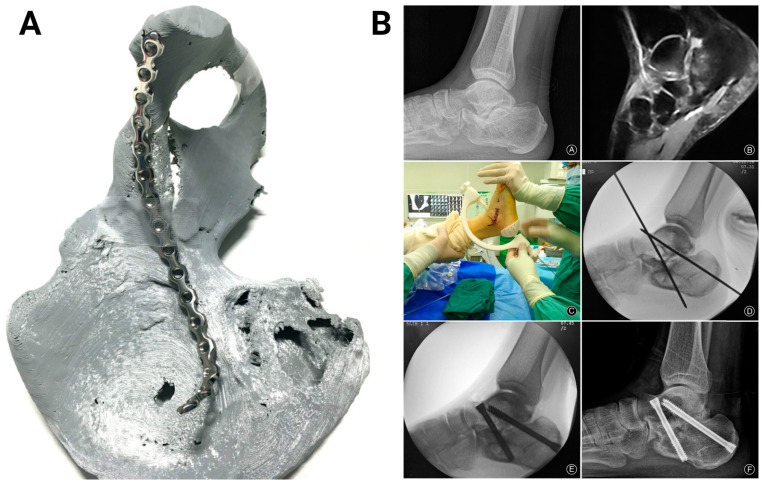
Three-dimensional-printed PLA-based surgical guides. (A) The 3D-printed acetabular fracture model served as a guide for pre-bending the steel reconstruction plate prior to surgery. The image was adapted with permission from Weidert et al. [100]. (B) A clinical case showing the subtalar joint space upon radiography (top left) and osteoarthritis upon magnetic resonance imaging (top right). The 3D-printed guide was used to position the Kirschner wires (middle). Intraoperative fluoroscopy confirmed the satisfactory placement of the wires (bottom left). After 1 year, radiography showed subtalar bony fusion and obliteration of subtalar joint space (bottom right). Images were adapted with permission from Duan et al. [105].
